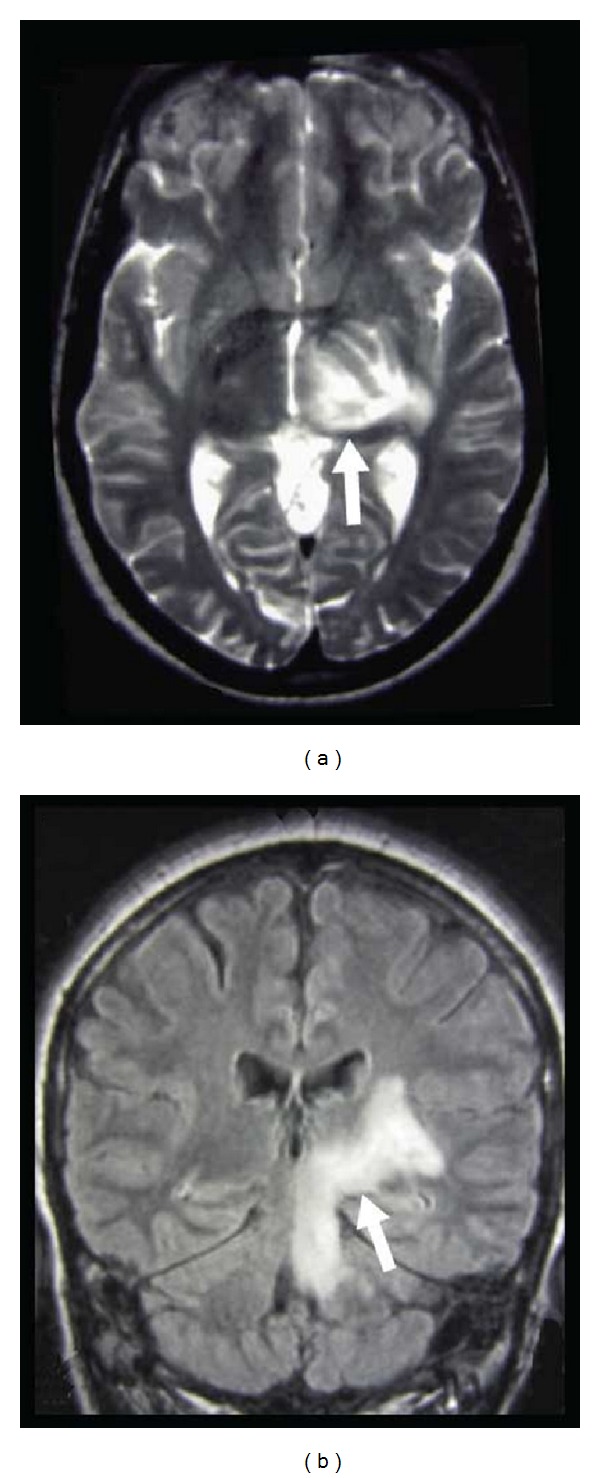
A patient with neuro-Behcet showing a left-sided hyperintense lesion on axial T2-weighted (a) and coronal FLAIR (b) imaging involving the cerebellum, the midbrain, and the inferior part of the thalamus (arrows), the internal capsule, and the putamen.
